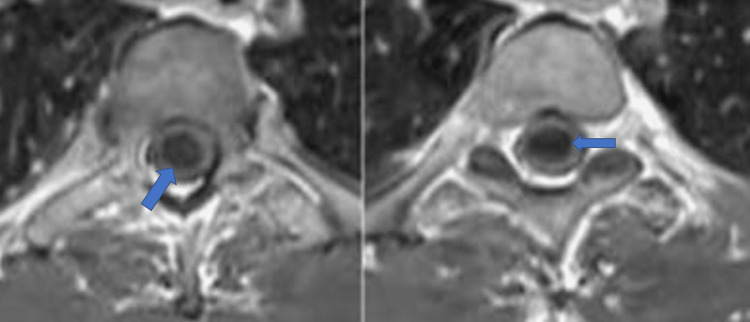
Preoperative MRI of the thoracic spine indicating the syrinx cavity Preoperative T1-weighted axial scan showing the syrinx cavity (blue arrows).
